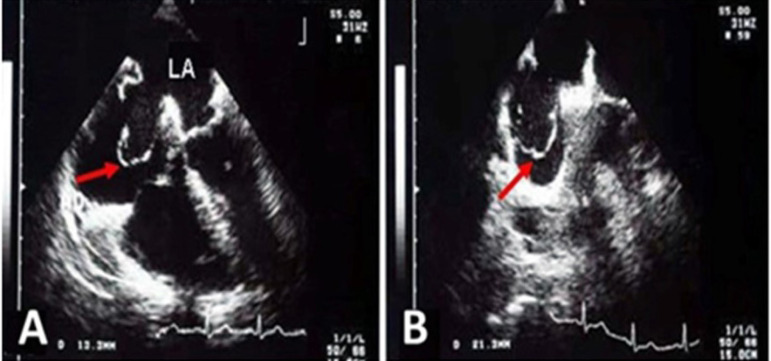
Transesophageal echocardiogram. A and B) The red arrow points to the atrial septal aneurysm. LA=left atrium.
